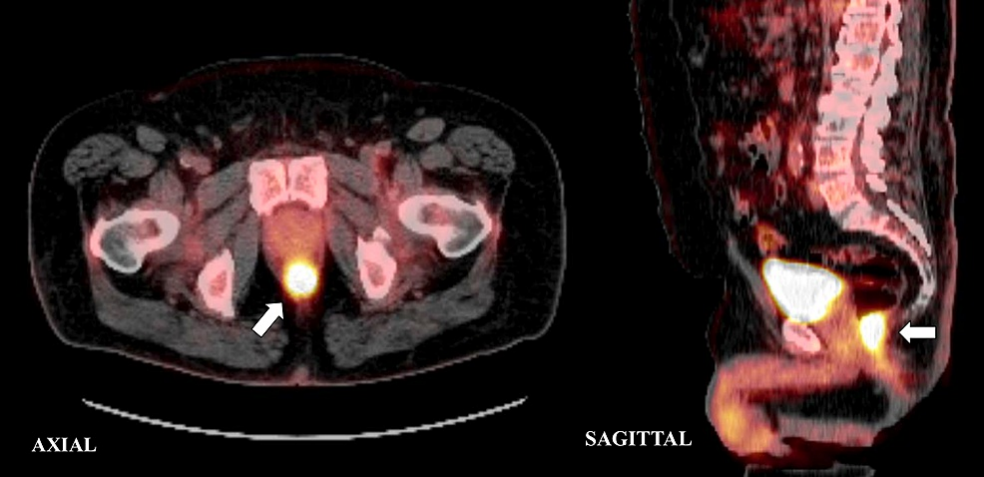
Axial and sagittal view of PET-CT scan demonstrating increased FDG uptake in the rectum. FDG: ^18^F-fluoro-2-deoxyglucose; PET-CT: positron emission tomography-computed tomography
